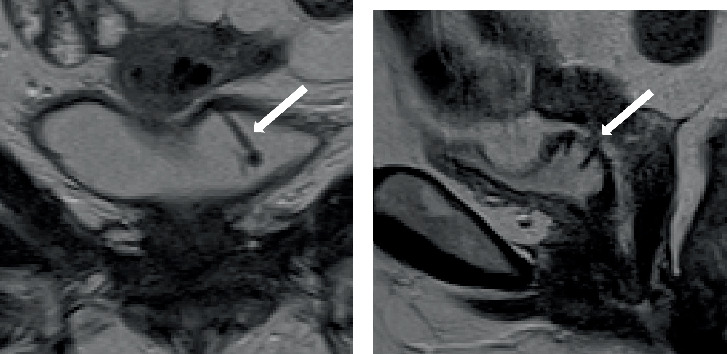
Preoperative MRI imaging demonstrating Lippes Loop IUD curls protruding into the bladder lumen in (a) coronal and (b) sagittal views (white arrows).
